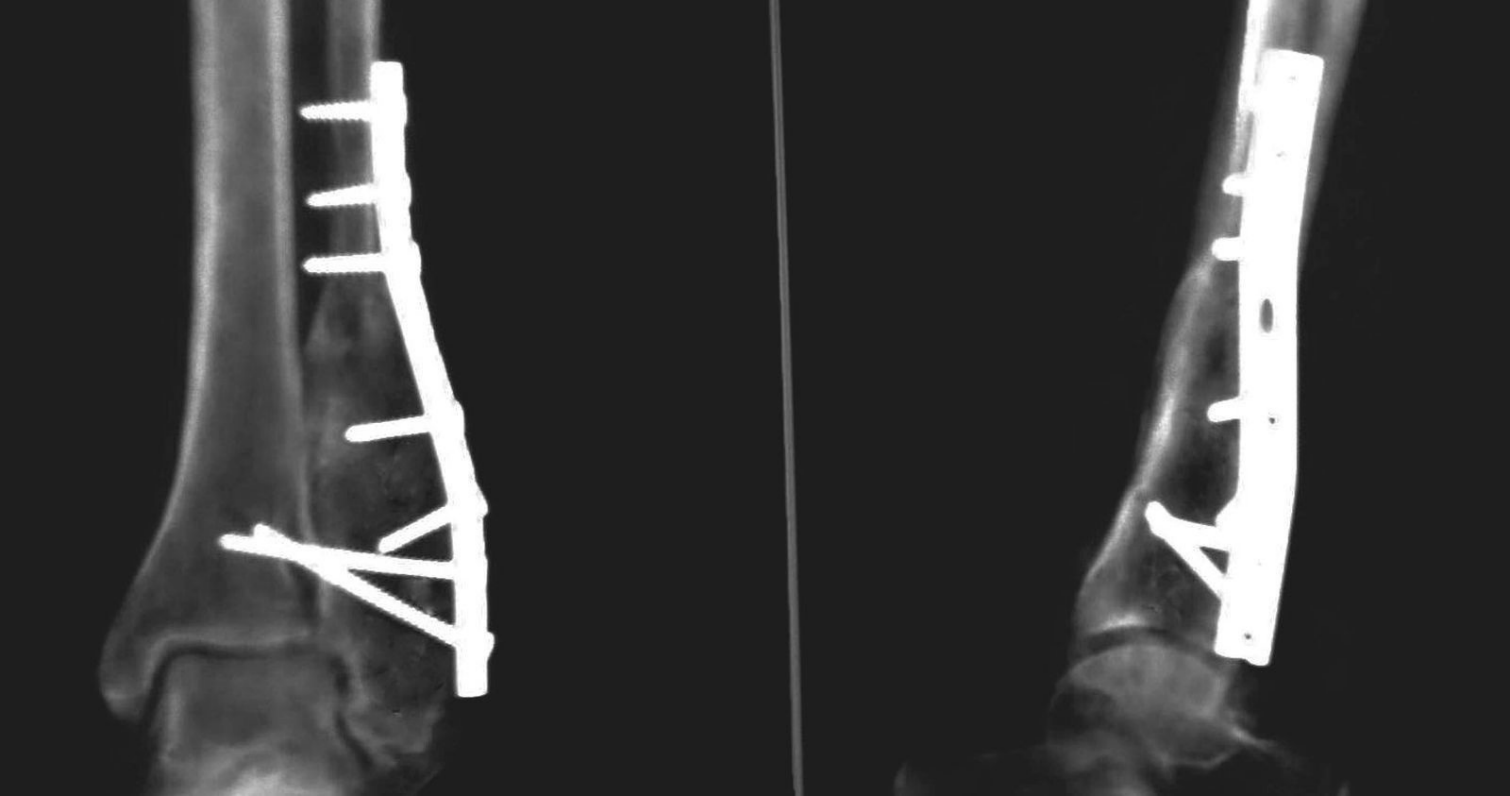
Plain radiograph (Anteroposterior and lateral view) showing 18 months follow-up case with incorporated bone graft
